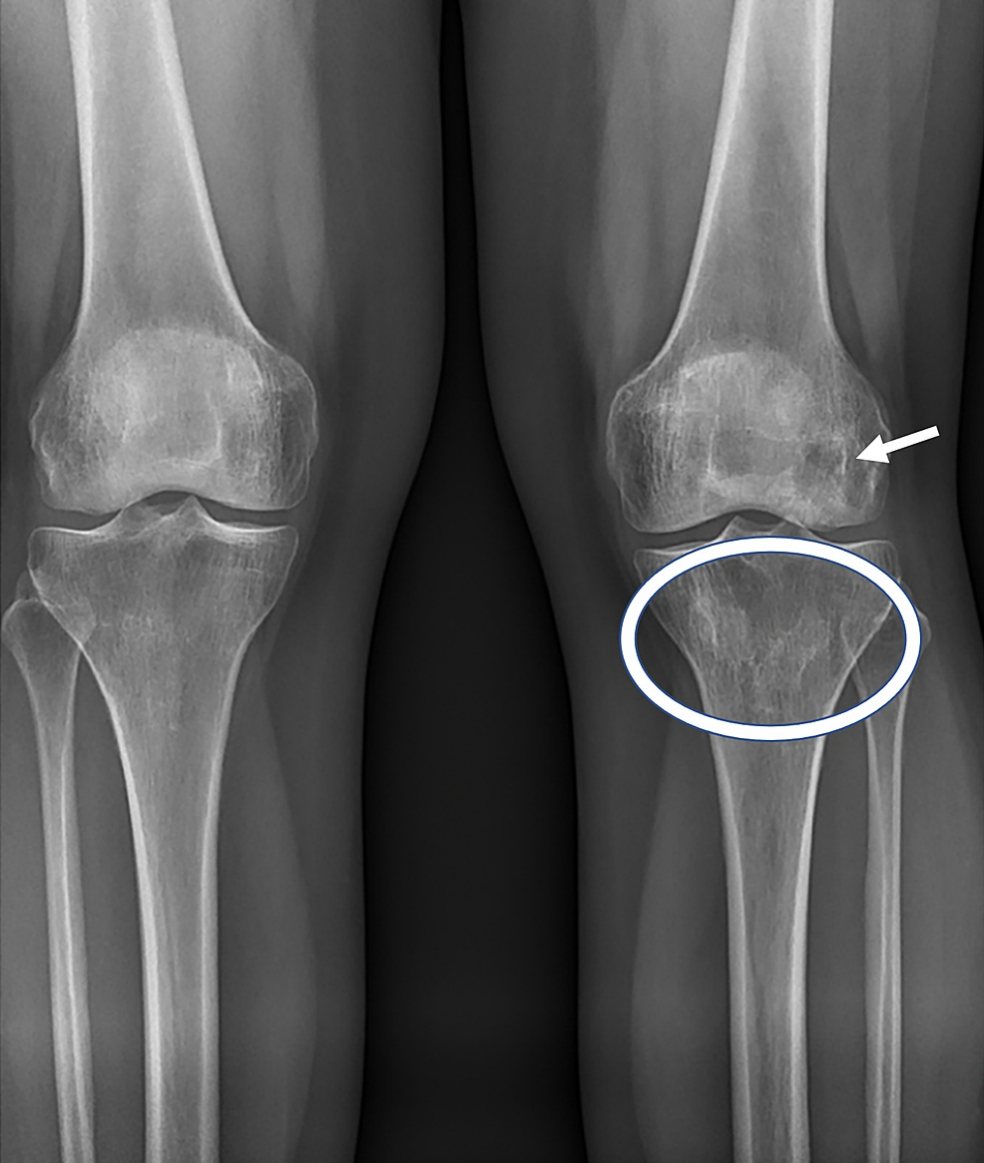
Anteroposterior radiographs of bilateral knees from September 16, 2019 The image demonstrates osteopenia and left distal femur (arrow) and proximal tibial (circle) areas of sclerosis, consistent with known bony infarcts
